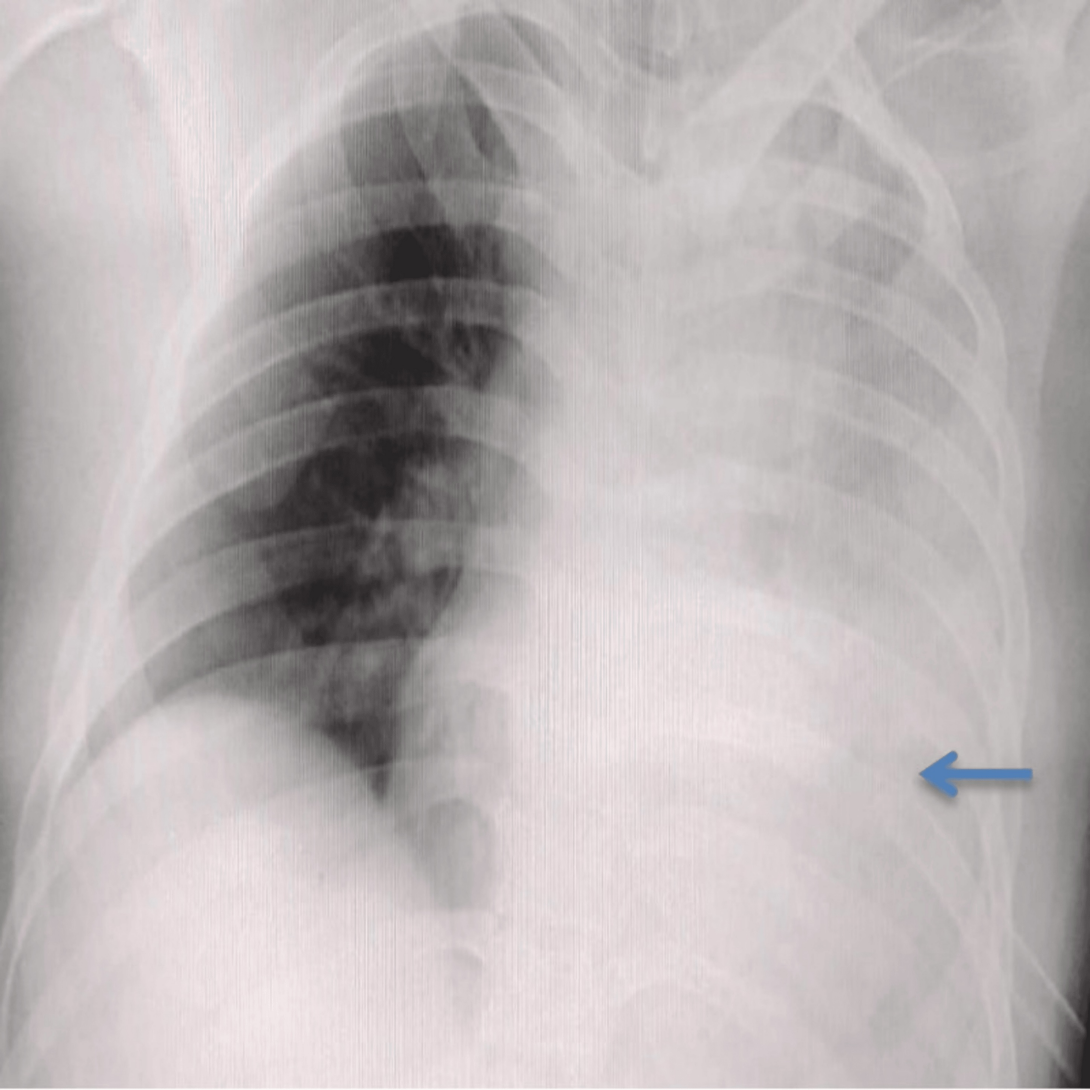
Chest X-ray (AP) obtained following clinical deterioration showing complete opacification (white-out lung) of the left hemithorax with the intercostal drainage tube in situ (arrow)
